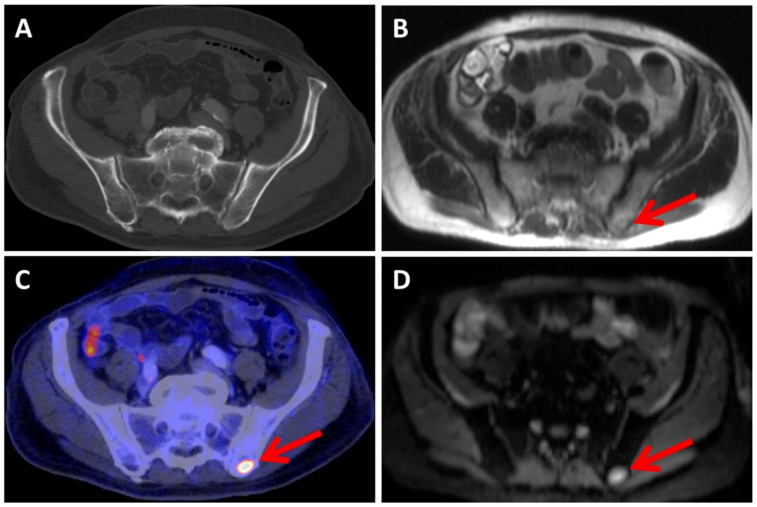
The patient presented with a metastatic bone lesion located in the left ilium (indicated by the red arrow), which is distinguishable in MRI using the HASTE sequence (B), DWI (D), and PET (C) imaging but is not visible in the CT scan (A).
